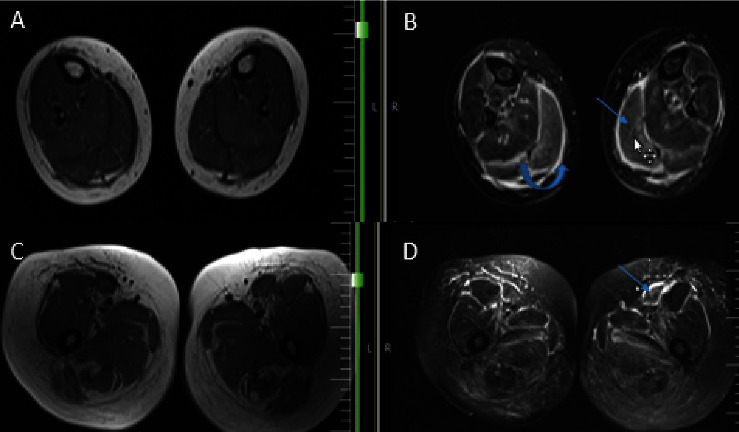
(a and b): MRI of both legs; T1W (a) and fluid sensitive fat sat (b) sequences; perimuscular and fascial edema (curved arrow) and intra muscular edema (arrow) within gastrocnemius muscles; (c and d): MRI of both thighs T1W (c) and fluid sensitive fat sat (d) sequences; perimuscular and fascial edema (arrow) more in anterior compartment.
